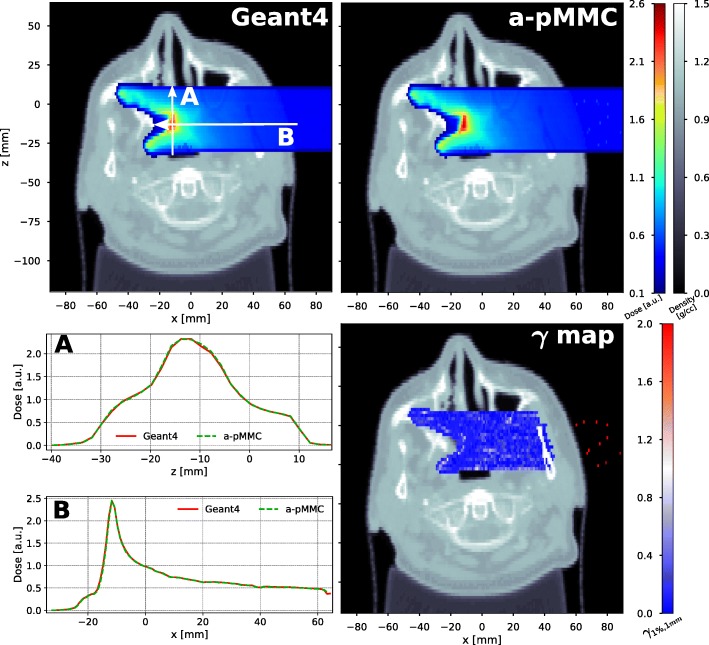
Results of the patient CT case. Transversal cut of the head and neck patient CT showing dose color washes for a mono-energetic proton 4×4 cm^2^ broad beam of 100 MeV calculated with Geant4 (top left) and the adaptive pMMC (top right). Dose profiles as indicated by the white arrows are shown (bottom left) and the result of a 3D-Gamma evaluation with a 1% (global) and 1 mm criterion (20% lower cutoff) is presented for the corresponding slice (bottom right)
